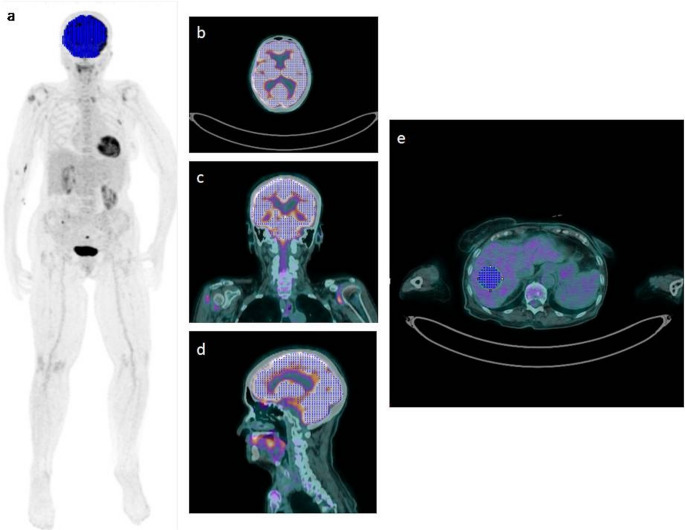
Image (a) shows the Maximum Intensity Projection (MIP) of the [¹⁸F]FDG PET scan, where the blue area represents the auto-segmented brain region used to calculate the mean SUV of this organ. The same segmented region is shown in the axial (b), coronal (c), and sagittal (d) planes. Image (e) shows the fixed spherical volume, in blue, used to measure the mean hepatic SUV in the right lobe
